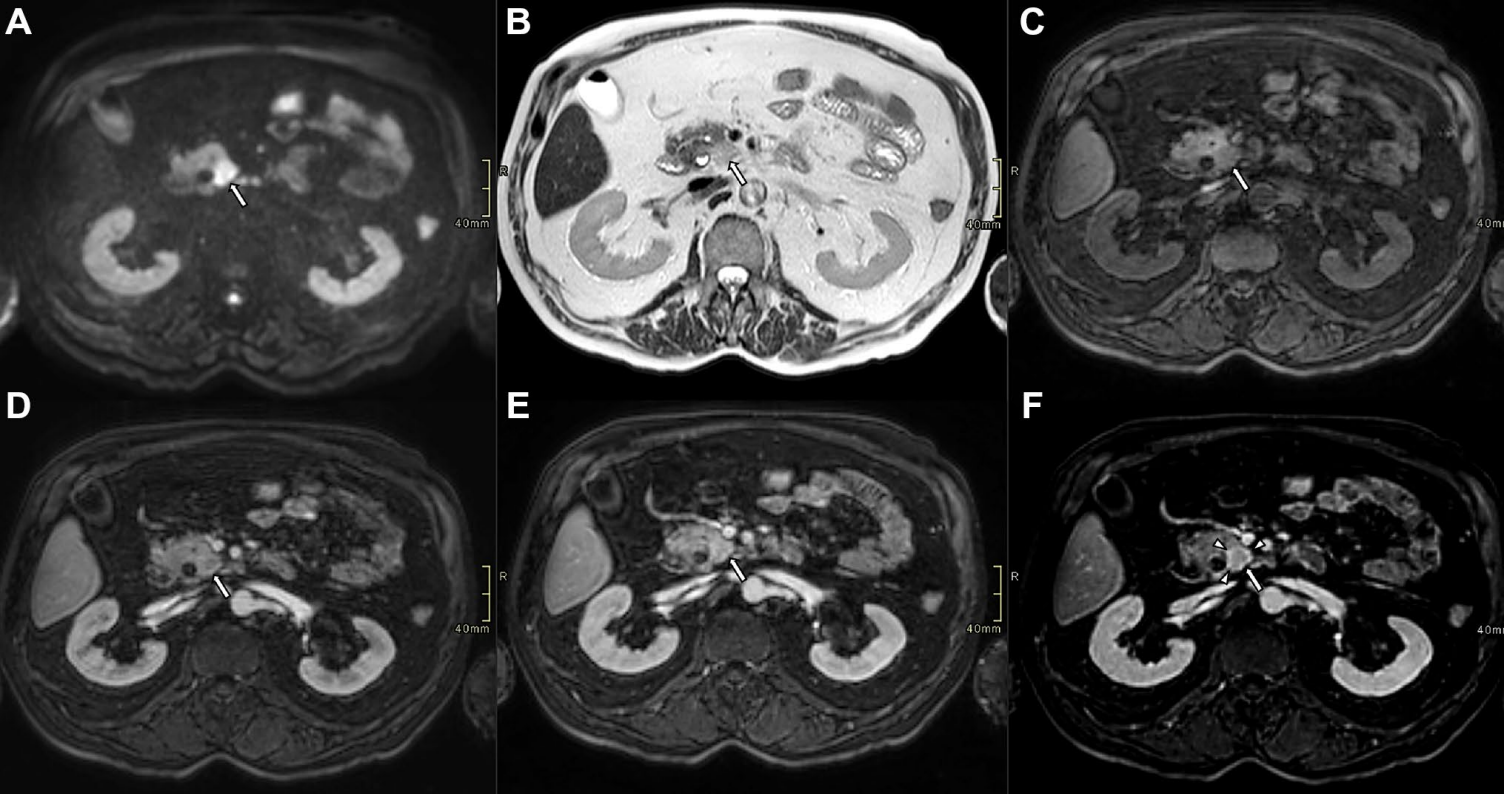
Axial contrast-enhanced MR images of a small pancreatic ductal adenocarcinoma at the pancreatic head (arrows). The tumor demonstrates strong signal restriction on Diffusion weighted image (A), hyperintensity on T2-weighted (B), and hyperintensity on T1-weighted (C) images. The lesion shows disomogeneous enhancement on pancreatic (D), and portal venous (E) phases. In the delayed phase (D), a well-defined enhanced rim (arrowheads) becomes visible, delineating the lesion margin more clearly than in the earlier phases
